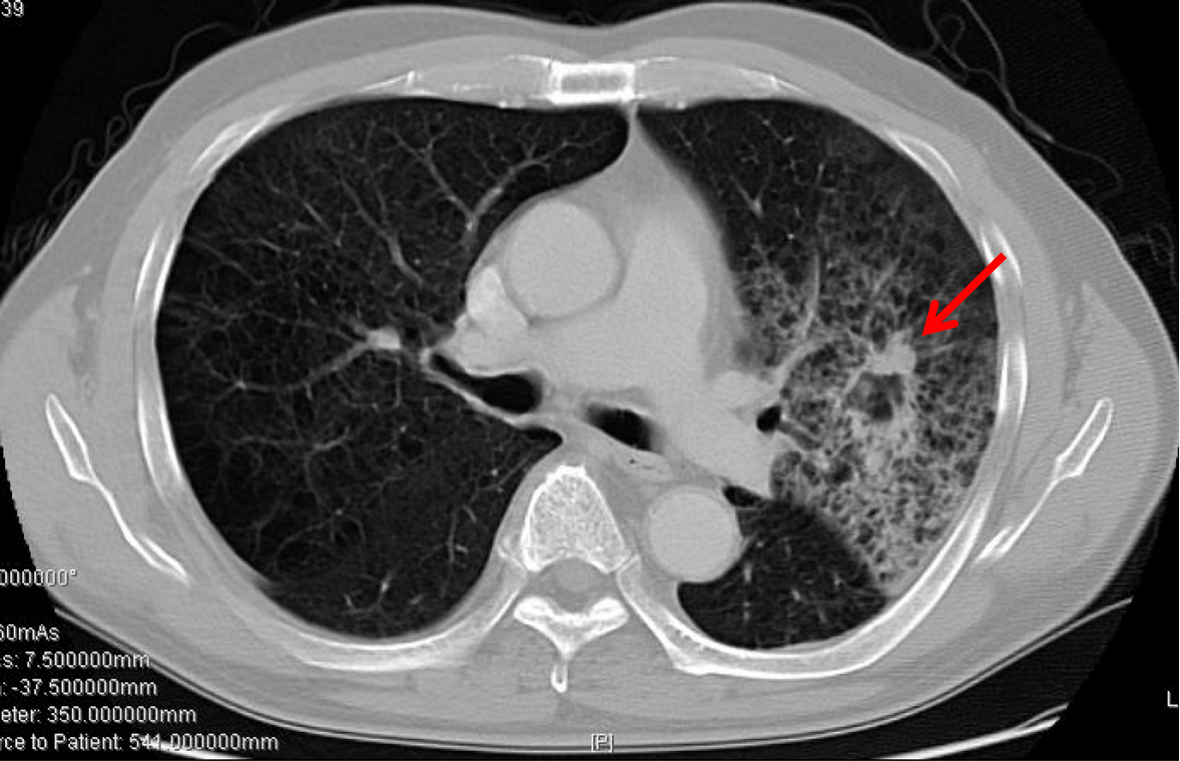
Chest computed tomography (CT) revealed a 28 × 18 mm spiculated cavitary nodule accompanied by parenchymal infiltration in the left upper lobe
